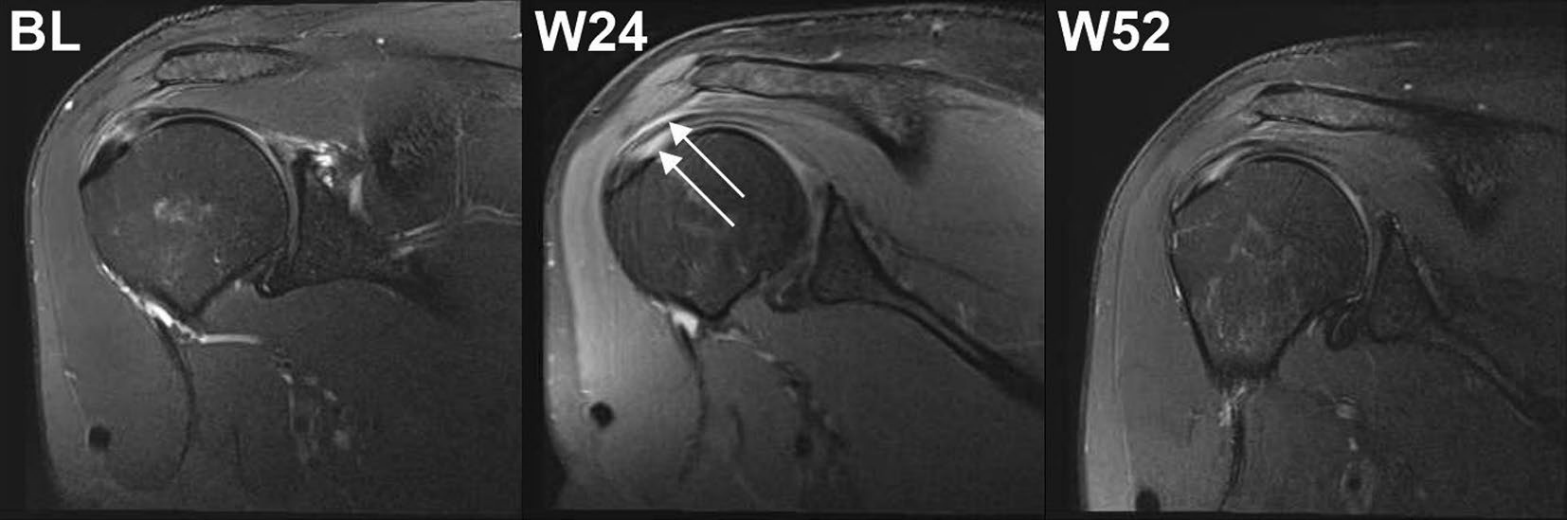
Proton density weighted, fat saturated, T2-weighted, coronal MRI scans of the index shoulder of Subject A4 (injection of UA-ADRCs), showing hyperintense structures at the position of the supraspinatus tendon at 24 weeks post-treatment (arrows) but not at baseline. *BL* baseline, *W24*/*W52* study visits scheduled in the former study^12^.
